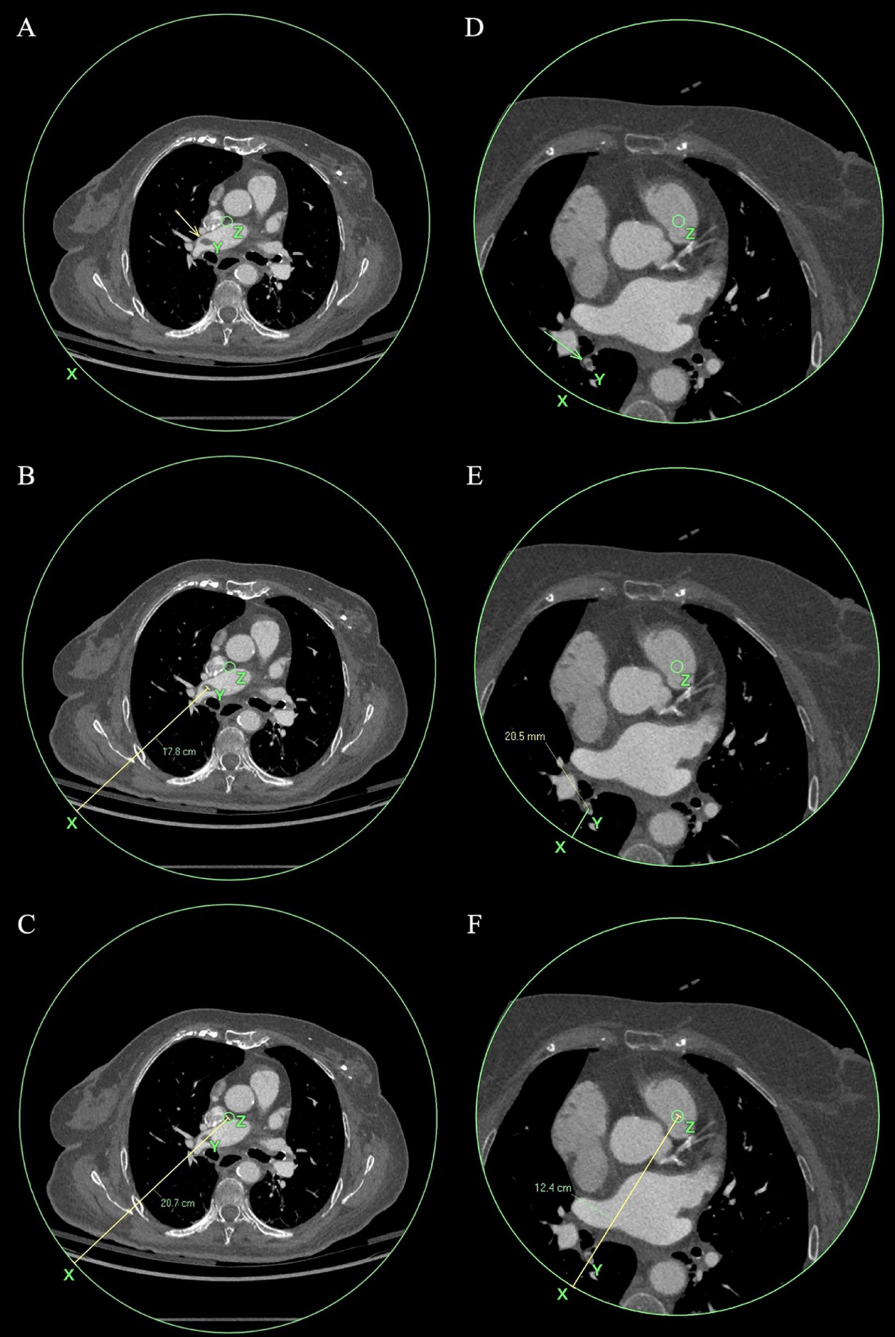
Measurement of Periphery Index in two cardiac CT angiography cases: Case 1: Transcatheter aortic valve implantation (TAVI) protocol in an 82-year-old female with pulmonary embolism (PE) in the right main pulmonary artery (images A-C). Case 2: Left atrial appendage (LAA) protocol in a 76-year-old female with subsegmental PE in the right lower lobe (images D-F). This protocol has a limited field of view (FOV), focusing on the heart. In each case: X marks the edge of the FOV, shown as a large green circle, Y marks the location of the PE (arrows in images A and D), Z marks the center of the FOV, shown as a small green circle. The Periphery Index is calculated as the ratio between the distance from the PE to the edge of the image (XY) and the radius of the FOV (XZ). Case 1 (images A-C): XY = 17.8 cm (image B), XZ = 20.7 cm (image C) -> Periphery index = 0.86. Case 2 (images D-F): XY = 20.5 mm = 2.05 cm (image E), XZ = 12.4 cm (image F) -> Periphery index = 0.17. A higher Periphery Index (closer to 1.0) reflects a more central PE in the image, while a lower index indicates a more peripheral location
